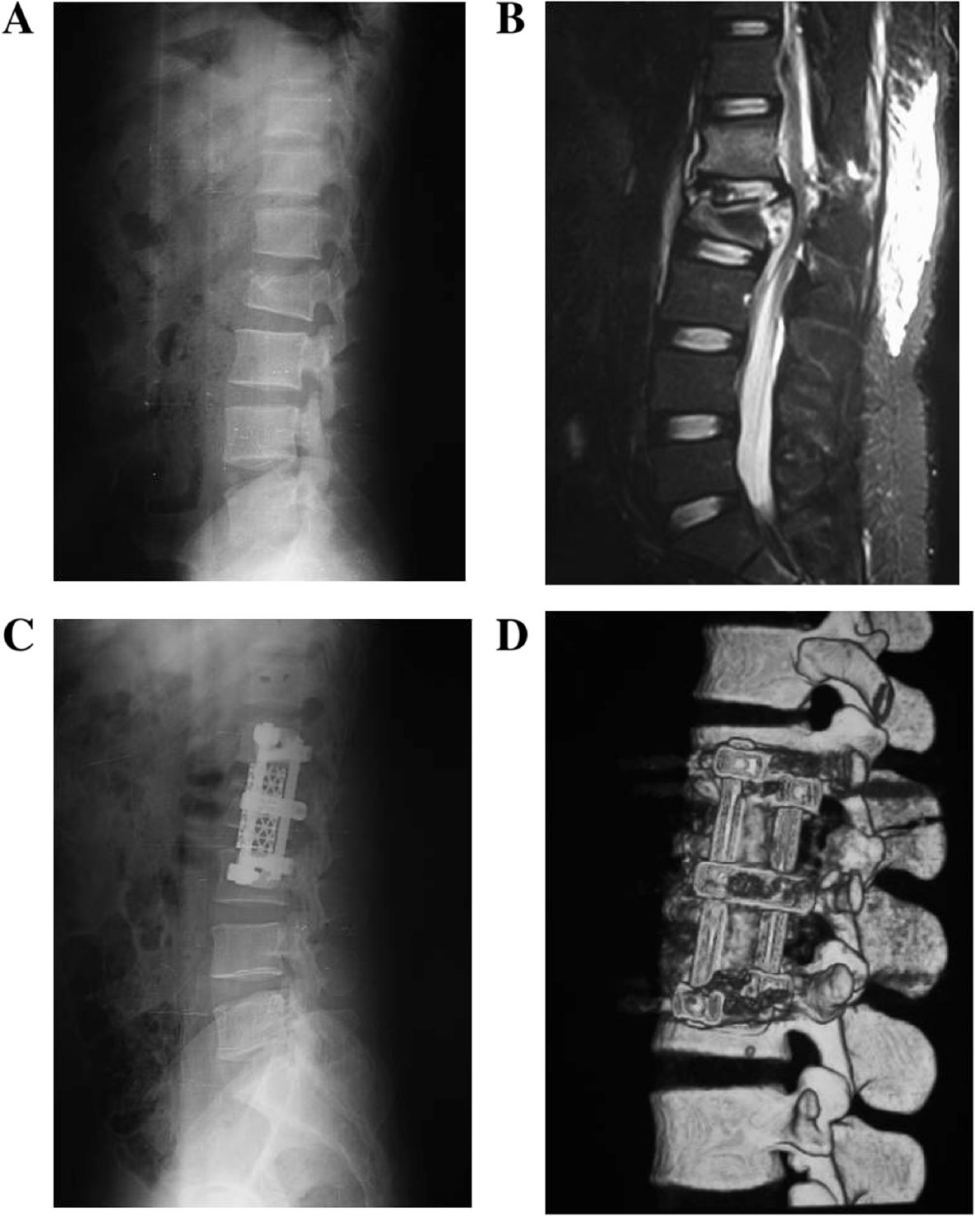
A 27-year-old man sustained a fall from ground level. (A) lateral x-ray shows burst fracture of L2. (B) MRI shows the burst fracture and canal compromise. (C) lateral x-ray and 3D-CT (D) show excellent alignment with the rods and screws in place.
